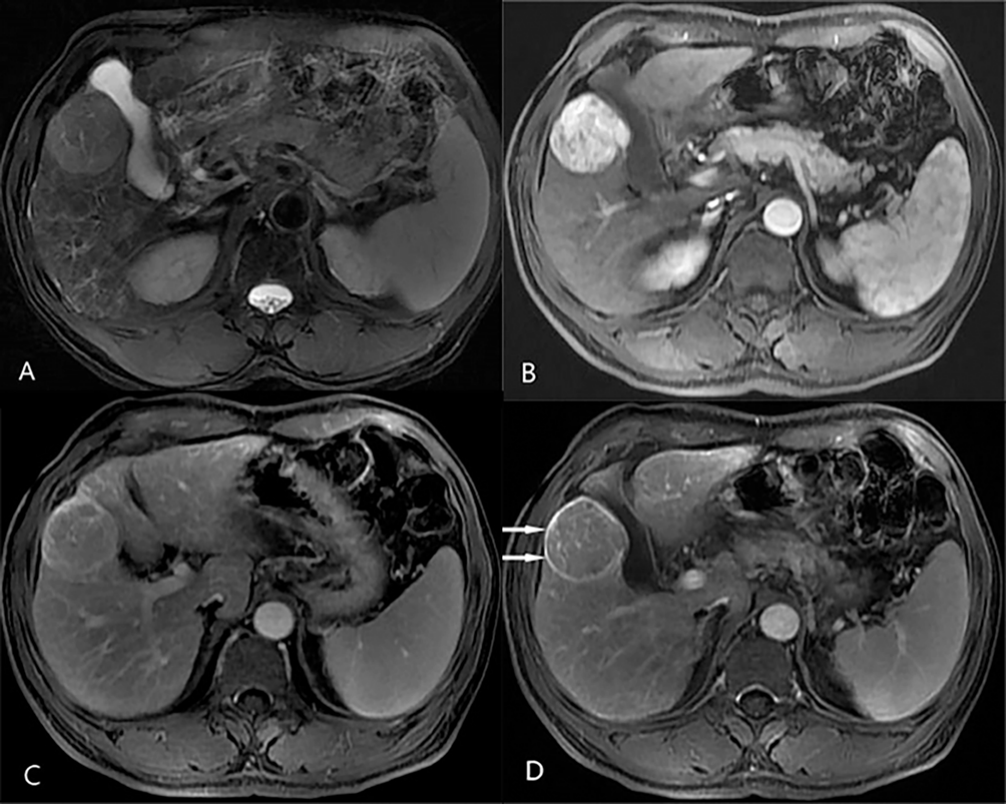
Axial MR images in a 63-year-old man with hepatitis B-related liver cirrhosis and hepatocellular carcinoma that satisfies the LR-5 criteria. (A) T2-weighted image shows a 49-mm nodule with mildly homogeneous hyperintensity in hepatic segment V. The nodule shows non-rim hyperenhancement in (B) the arterial phase and nonperipheral washout in (C) the portal venous phase. An enhancing capsule is also observed in (D) the delay phase.
